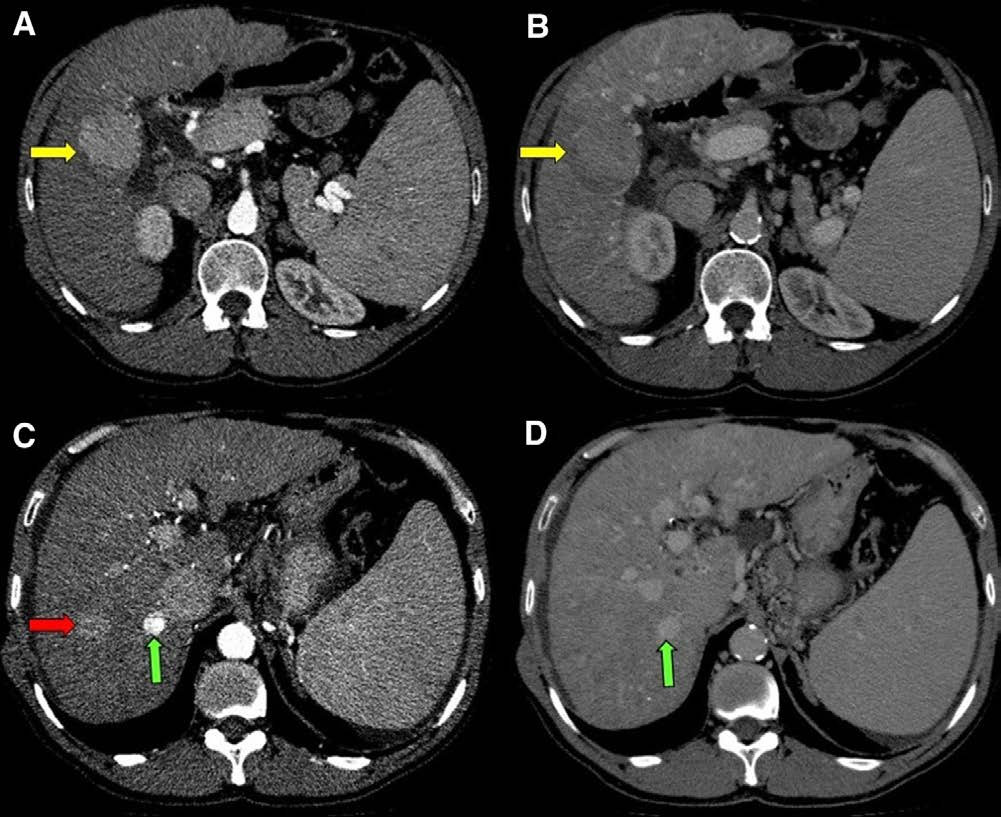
Cross-sectional computed tomography of the abdomen. In segment V of the liver, there was a contrast-enhanced nodule in the arterial phase (A, yellow arrow) with washout in the portal phase (B, yellow arrow), measuring 48mm in largest diameter (LI-RADS 5). In segment VII, another contrast-enhanced nodule in the arterial phase was evidenced (C, red arrow) with no typical washout in the portal phase (D), measuring 25mm in largest diameter (LI-RADS 4). There was also a nodule measuring 14mm in segment VII with arterial enhancement (C, green arrow) and high density in the portal phase (D, green arrow), which was previously characterized as hepatic hemangioma (LI-RADS 1).
